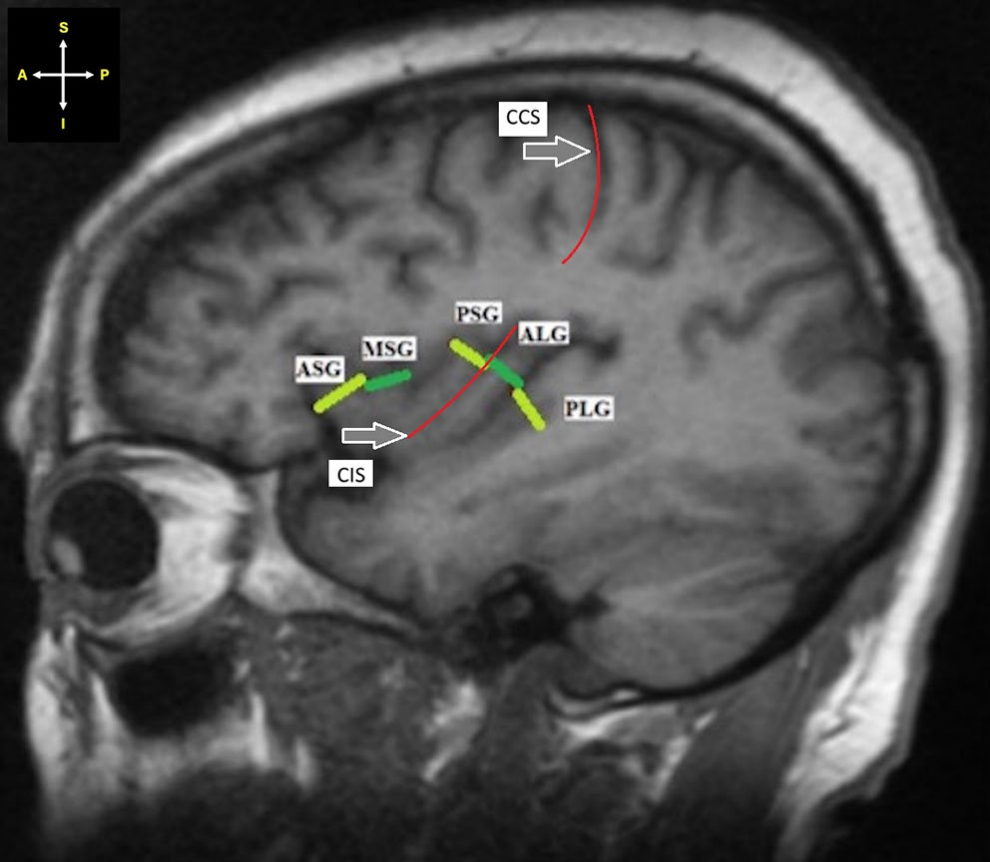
Sagittal section of MRI showing the location of the insular gyri in relation to the CIS. *Key* CCS – Central cerebral sulcus; CIS – Central insular sulcus; ASG -Anterior short insular gyrus; MSG - Middle short insular gyrus; PSG - Posterior short insular gyrus; ALG - Anterior long insular gyrus; PLG - Posterior long insular gyrus
